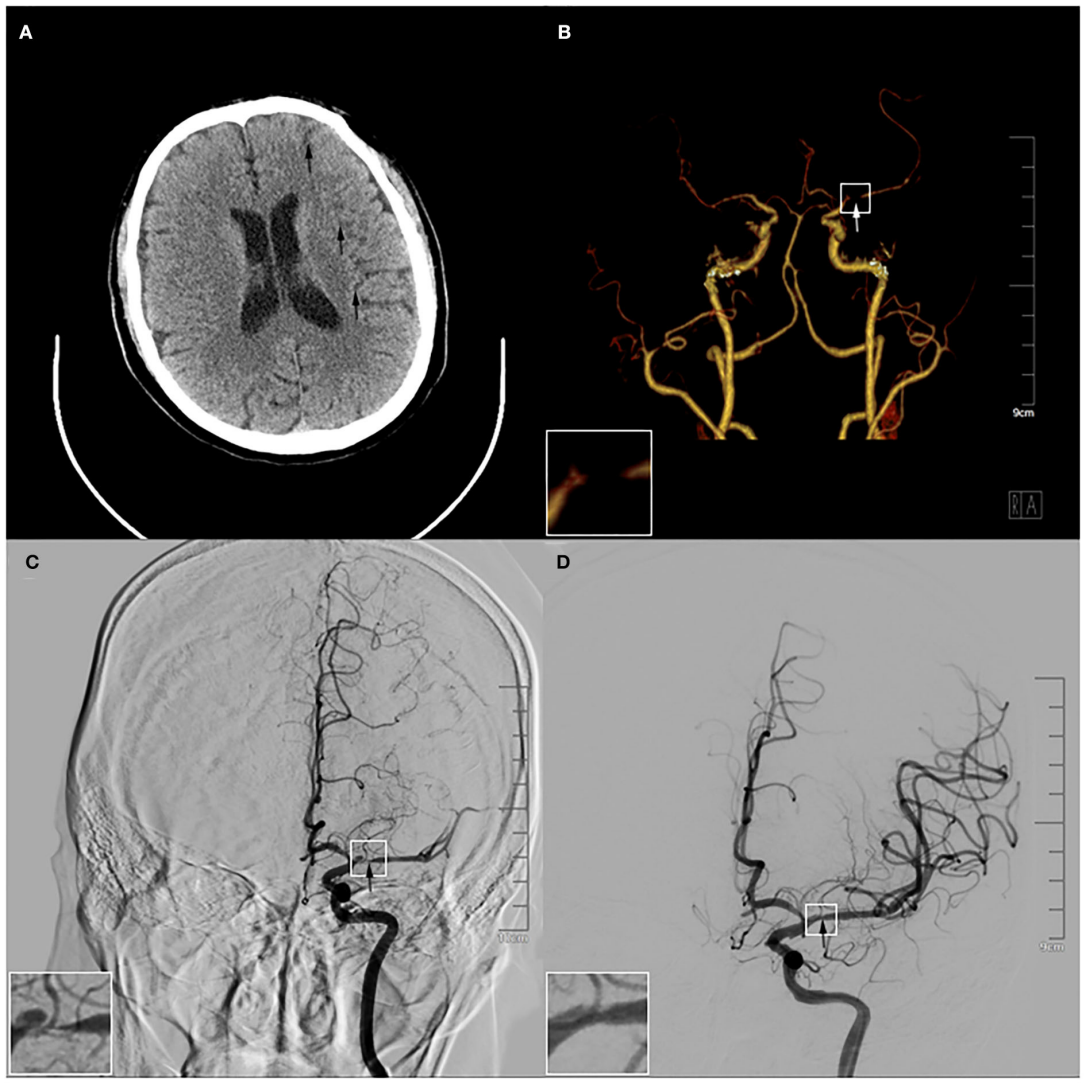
Results of preoperative and intraoperative imaging examination. (A) The brain computed tomography showed multiple pieces of low-density signal were in the left cerebral hemisphere (arrows). (B) The computed tomography angiography exhibited the M1 segment of the left middle cerebral artery (LMCA) was partially interrupted (arrow). (C) The angiography before the operation displayed that there was stratification in the M1 segment of the LMCA (arrow). (D) The angiography after the operation demonstrated the stratification disappeared after the 3.5*20 mm intracranial self-expanding stent had been released in the LMCA and the vascular morphology was significantly improved (arrow). The magnification of the white box were in the left lower corner of (B–D).
